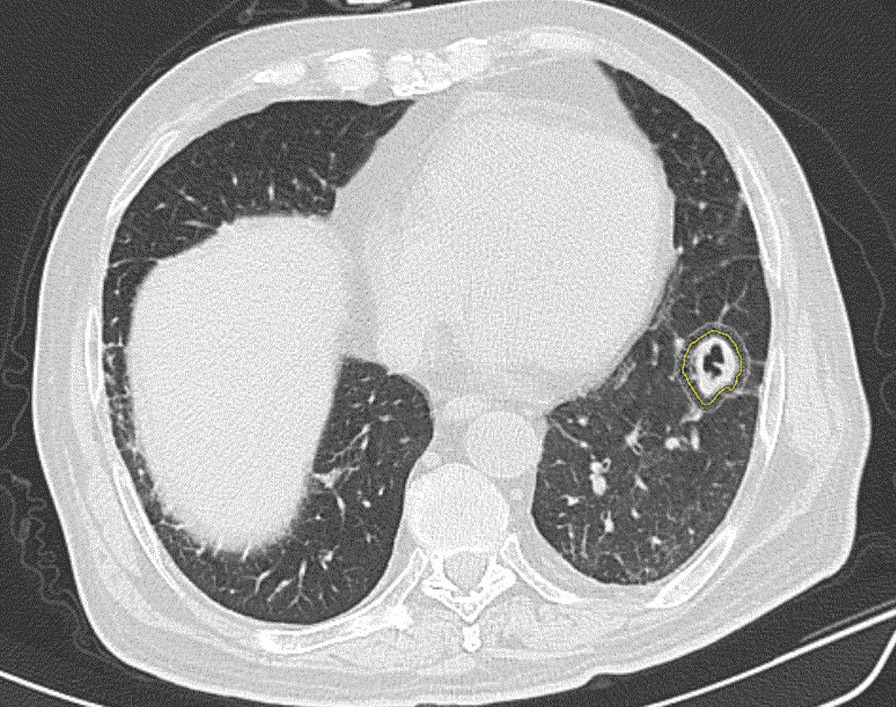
A 42-year-old man with AIDS and invasive aspergillosis presented with a 3-week history of expectoration. CT showed a thin-walled cavity lesion in the lower lobe of the left lung. The ground-glass opacity component was observed in the right outer edge of the lesion, and the radiologist manually adjusted the lesion edge after automatic machine delineation to include the GGO
